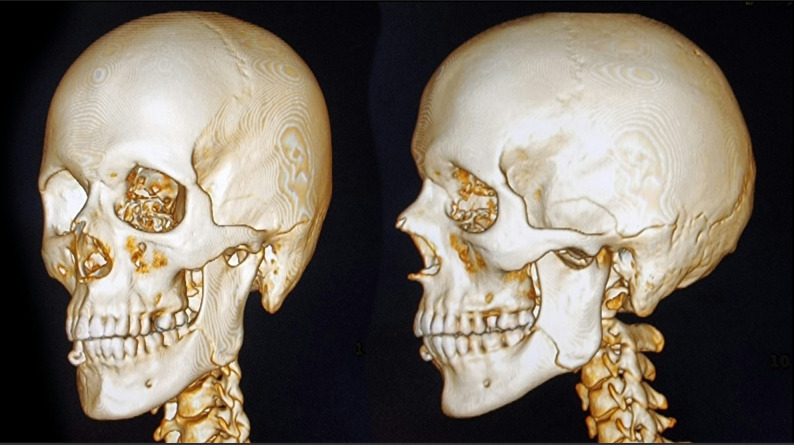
3D window of preoperative CT view showing left mandibular angular fracture.
